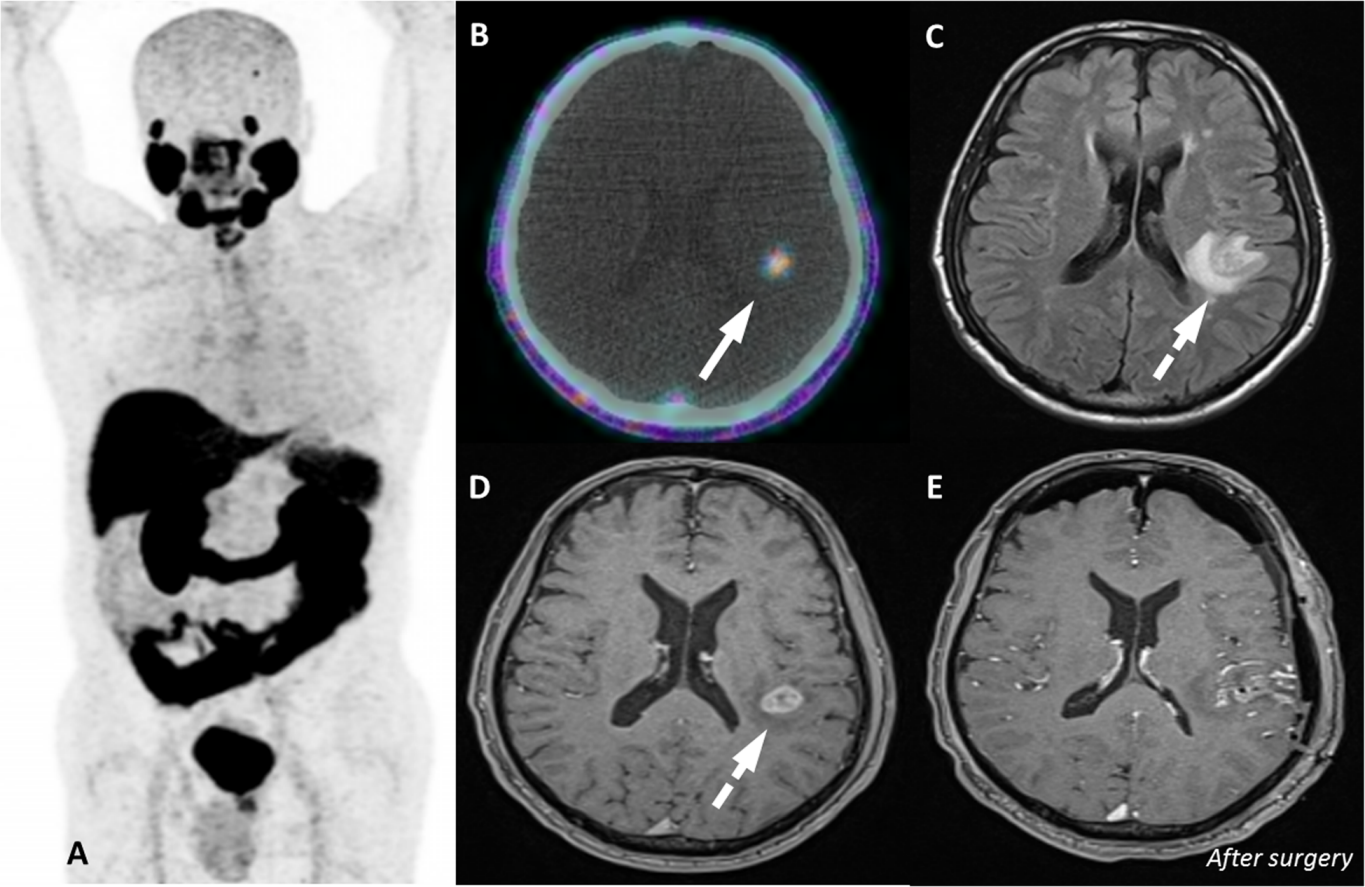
Glioblastoma multiforme with PSMA uptake. ^68^Ga-PSMA PET MIP (a), axial PET/CT (b), axial FLAIR (c) and T1w after gadolinium (d) images show a mild uptake in a cortico-subcortical lesion on the left parietal lobe (arrows) corresponding to a necrotic lesion with anelar enhancement and adjacent vasogenic edema (dashed arrows), confirmed as a Glioblastoma after surgical resection (e)
